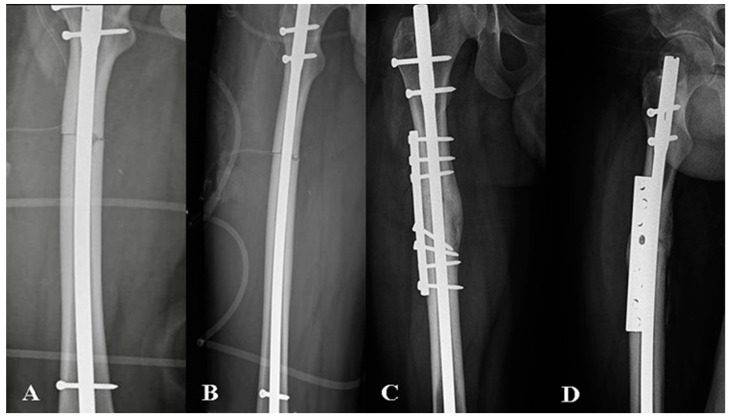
Radiological images depicting a 21-year-old patient with atrophic nonunion of the femoral shaft who underwent plate augmentation with an autologous bone graft. The images included preoperative radiographs: (A) anteroposterior radiograph and (B) lateral radiograph, as well as postoperative follow-up; (C) anteroposterior and (D) lateral radiographs.
